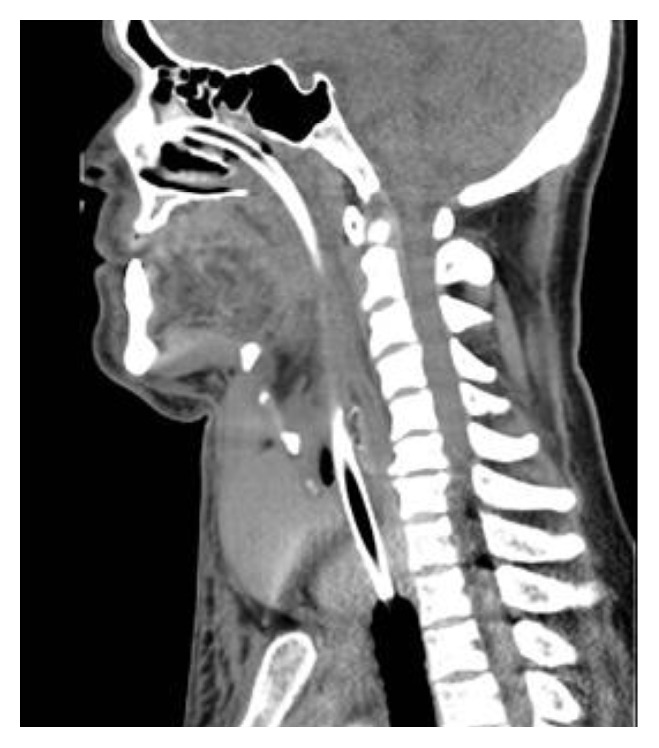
Arterial phase CT neck: parasagittal view of neck haematoma.
